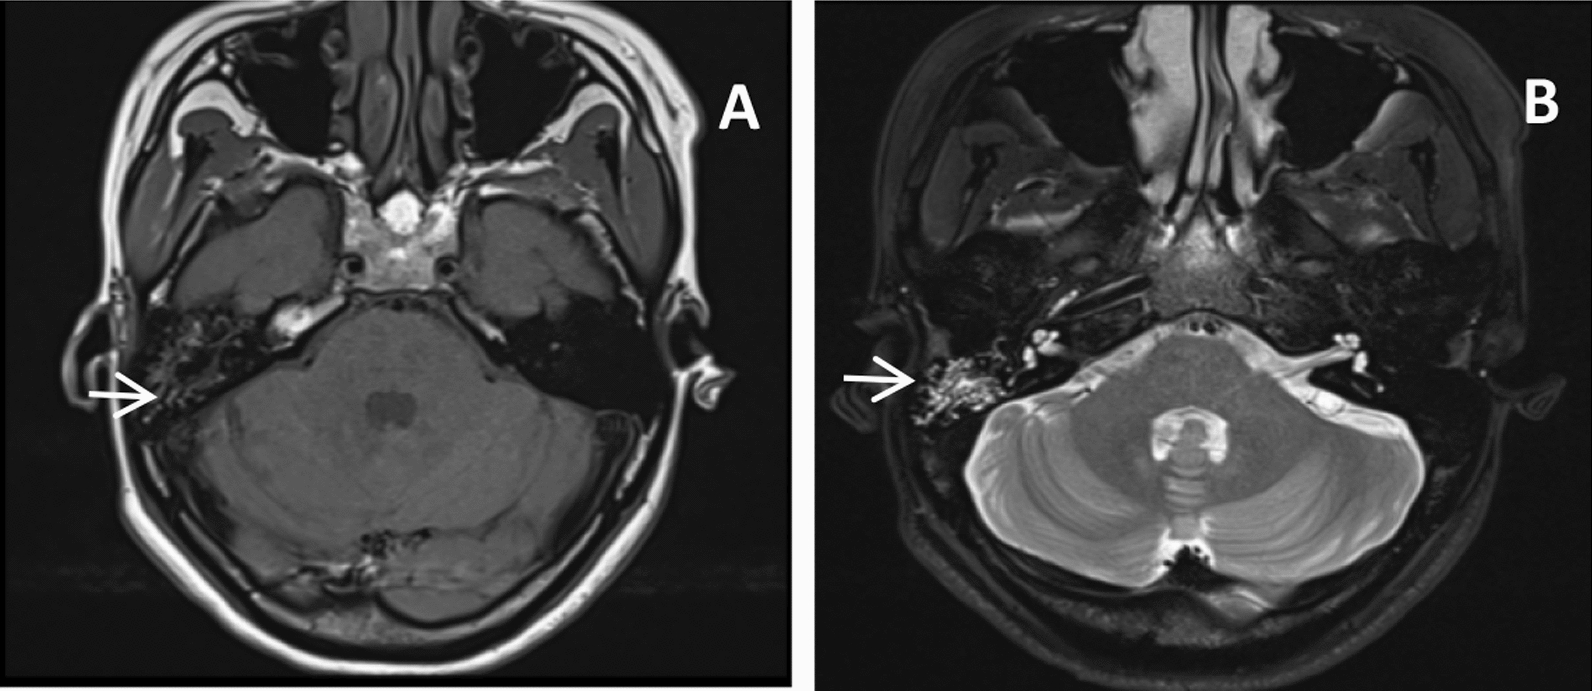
Pretreatment magnetic resonance imaging findings of right mastoid pathology. A T1-weighted imaging reveals a hypointense signal (arrow) within right mastoid air cells. B Corresponding T2-weighted image shows a hyperintense signal (arrow), indicative of fluid content. Inner ear structures, the cerebellopontine angle, and the brainstem maintain normal signal intensity
